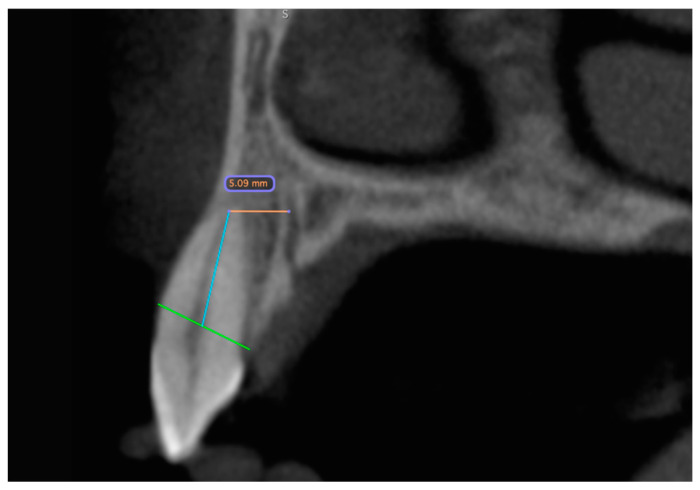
CBCT dataset with the distance measurement of the accessory canal to the neighboring tooth shown in the sagittal slice. The green line represents the bone level. Starting from this, a blue line is drawn along the wall of the pulp cavity to the apex of the tooth. At its end, it forms an endpoint of the orange measurement section. The distance is measured from here to the outer edge of the canal.
